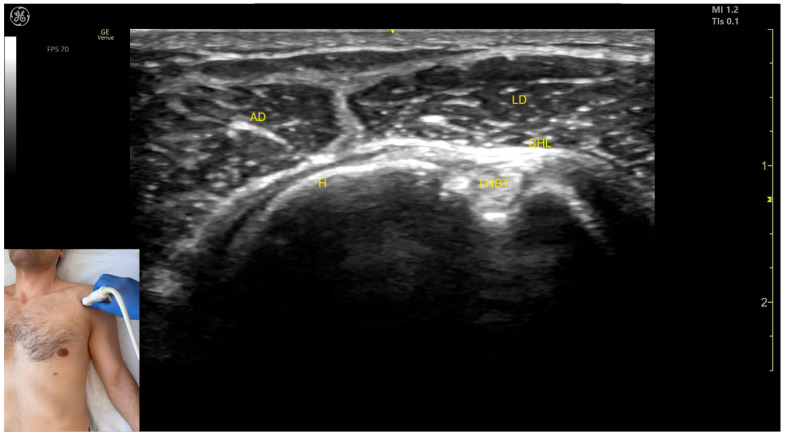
Ultrasound anatomy of the anterior deltoid (AD) with key landmarks: AD—anterior deltoid; LD—lateral deltoid; H—humerus; LHBT—long head bicep tendon; and CHL—coracohumeral ligament.
